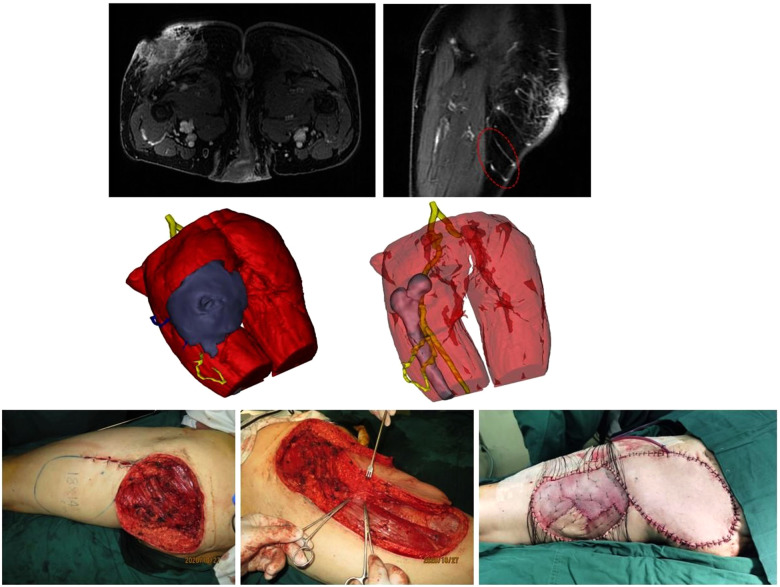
(Above) preoperative MRI showing the tumor infiltrating the underlying muscle (left) and adjcent perforators (right). Red dotted line circling the preferred perforators for PPPF. (Center, left) 3DR showing the surgical anatomy involving the tumor, underlying muscle, and perforators. The PPPF was planned based on the yellow perforators. (Center, right) The yellow perforators were intact after wide excision of the tumor. (Below, left) the wound after *en bloc* tumor resection and the flap design. (Below, median) harvesting the flap. The clamps pointed to the perforators for PPPF. (Below, right) rotation of the flap for 160° to cover the wound and free skin graft to close the donor site.
